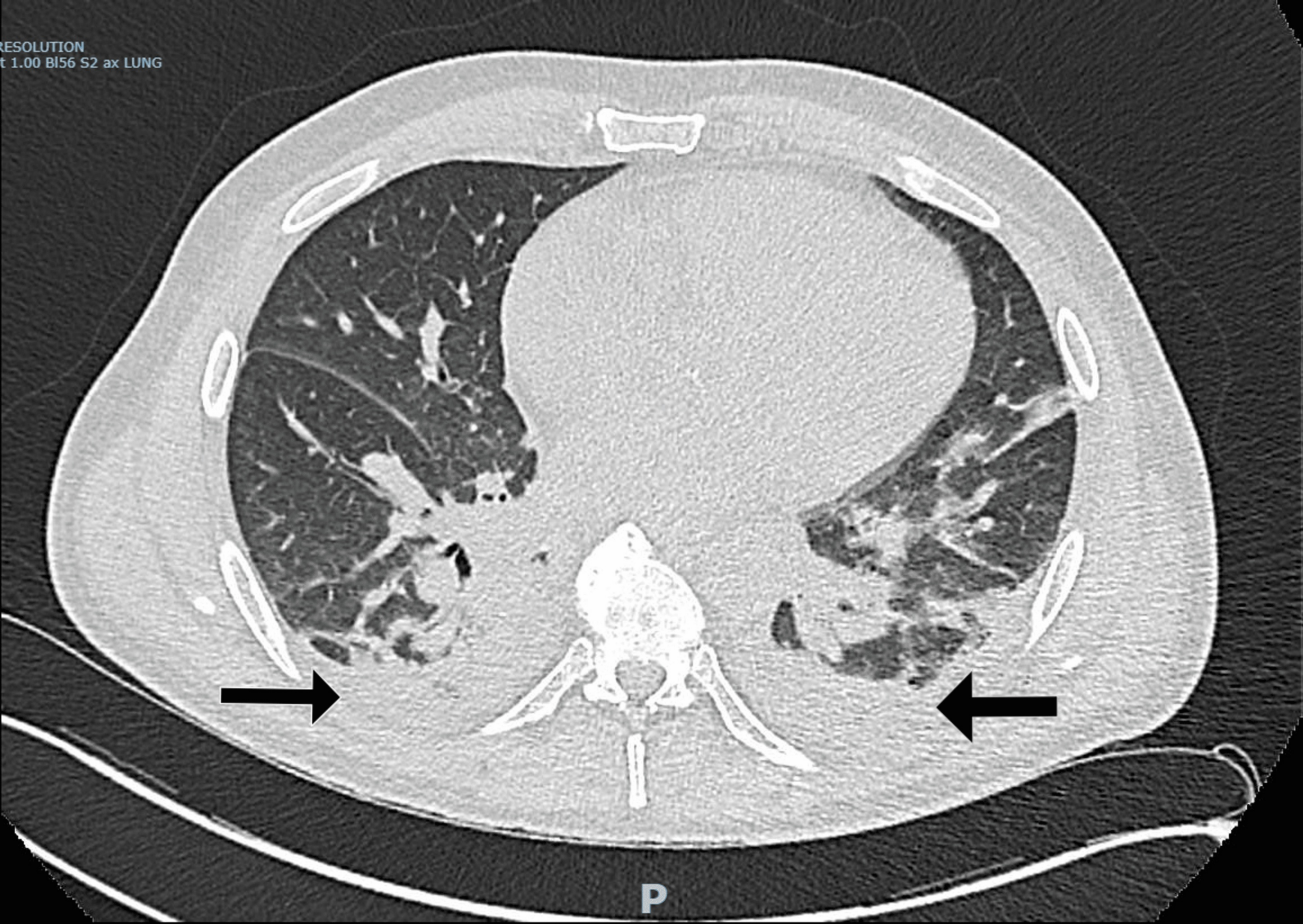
CT showing peribronchovascular opacification and bilateral pleural effusions. Note the arrows pointing towards effusions and opacifications
